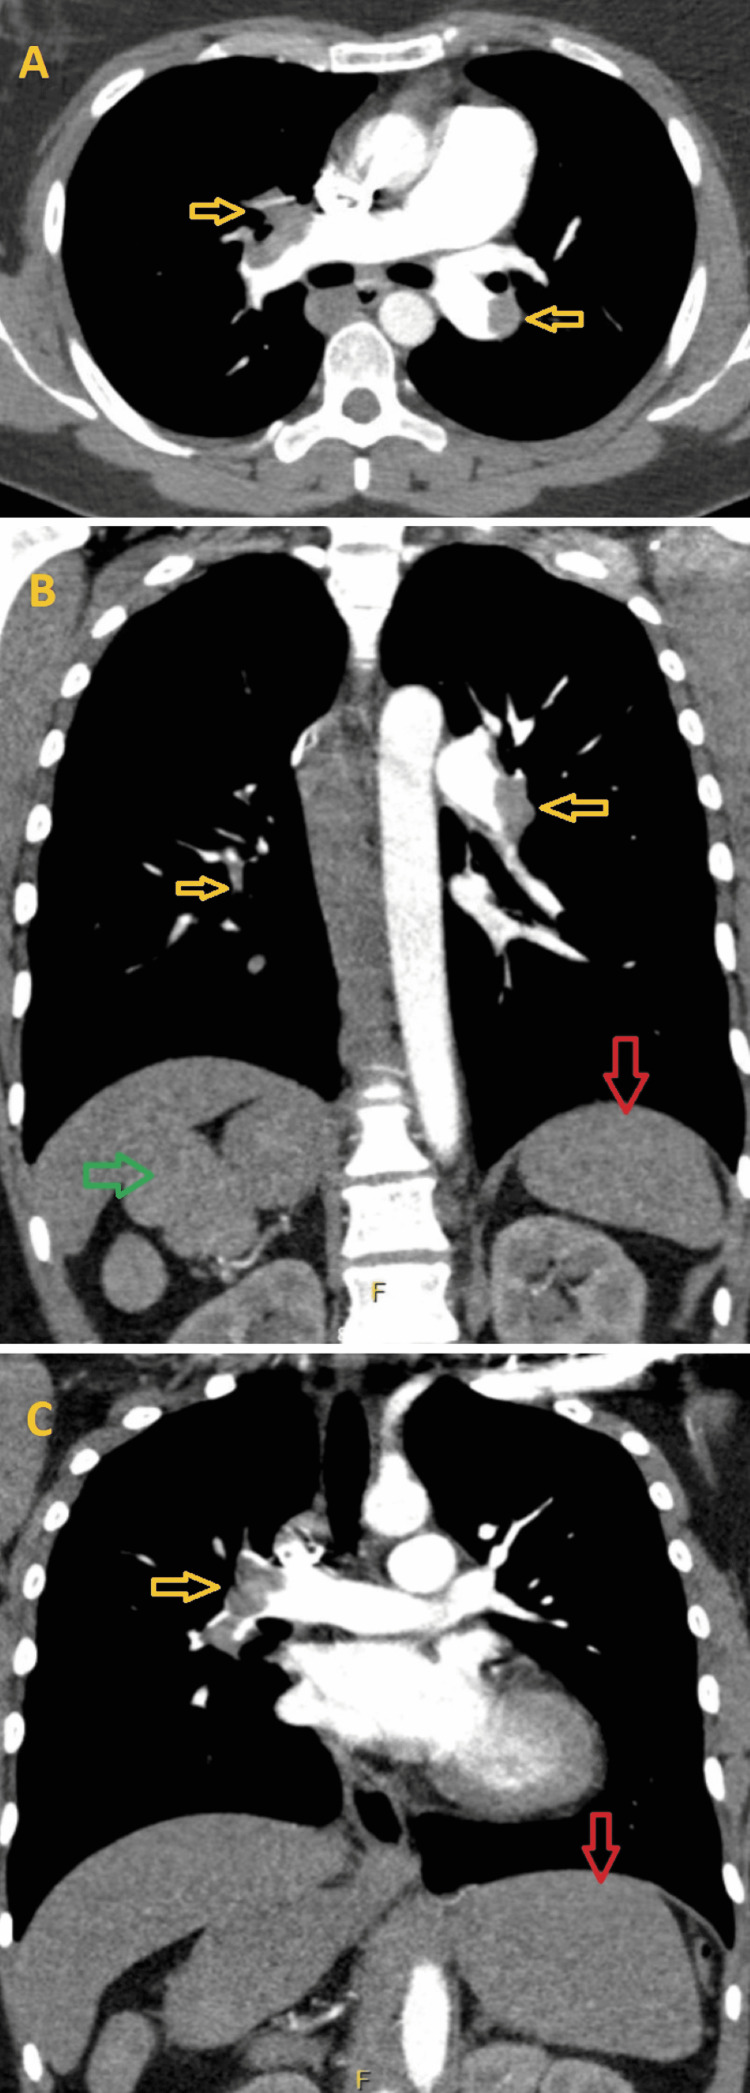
(A) Axial view and (B, C) coronal view of CTPE showing bilateral main pulmonary arterial emboli extending to the lobar and segmental branches (yellow arrows), right-sided polysplenia (green arrow), and a prominent left hepatic lobe (red arrows). CTPE - CT pulmonary embolism study
